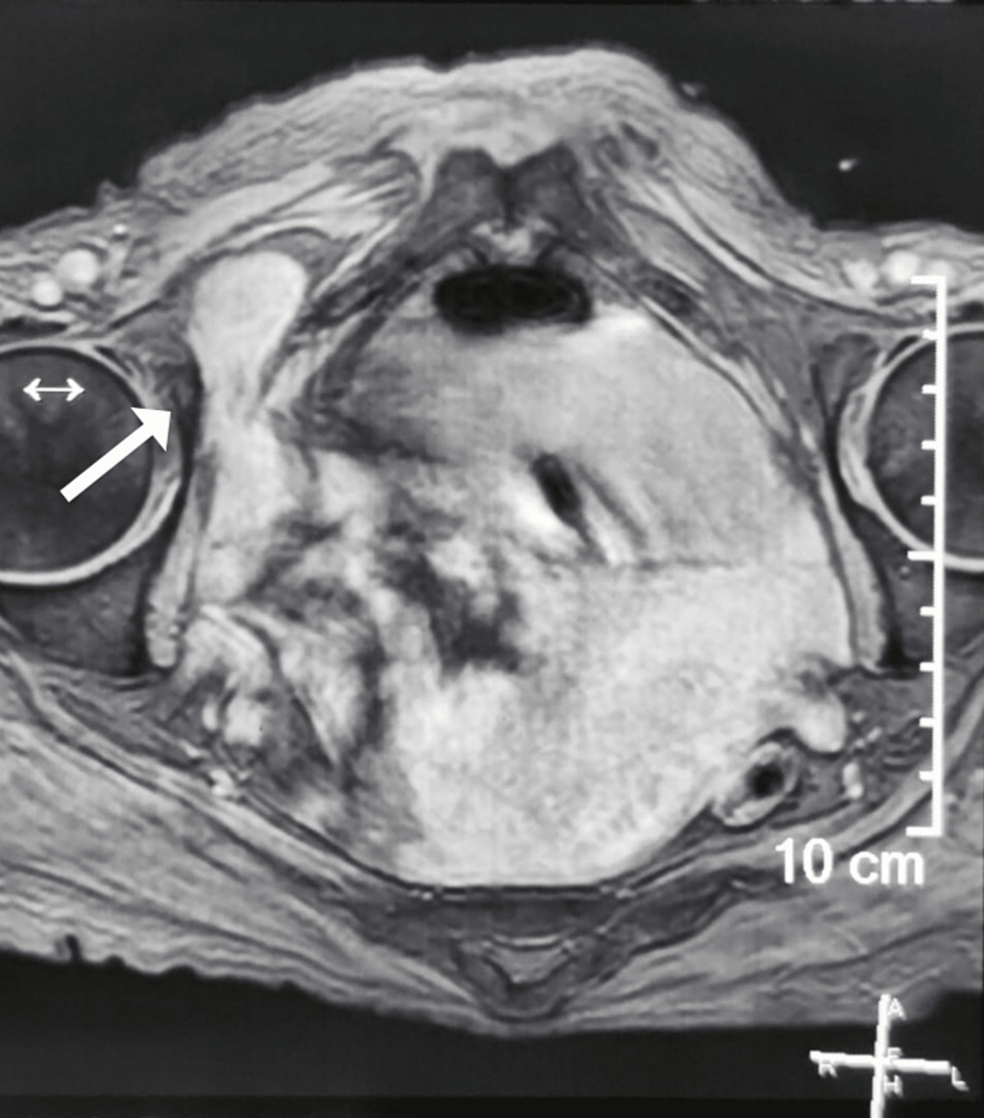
Magnetic resonance imaging: arrow showing bowel loop in right obturator canal.
